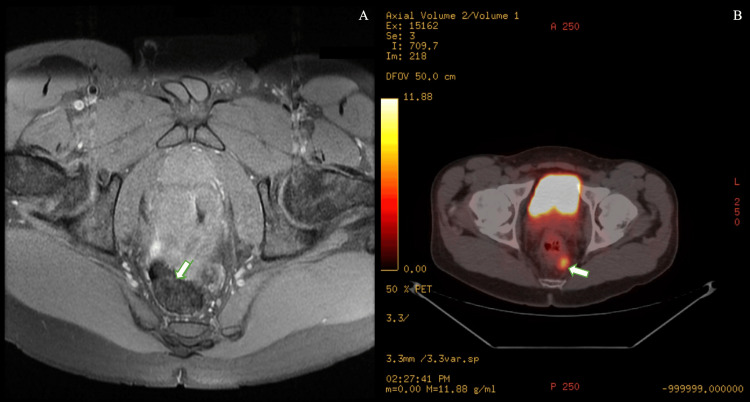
A) A 1.7 cm enhancing soft tissue lesion in the left supralevator space adjacent to the rectosigmoid junction. B) A 1.8 cm hypermetabolic left perirectal lymph node (maximum SUV=7.6). SUV, standardized uptake value
